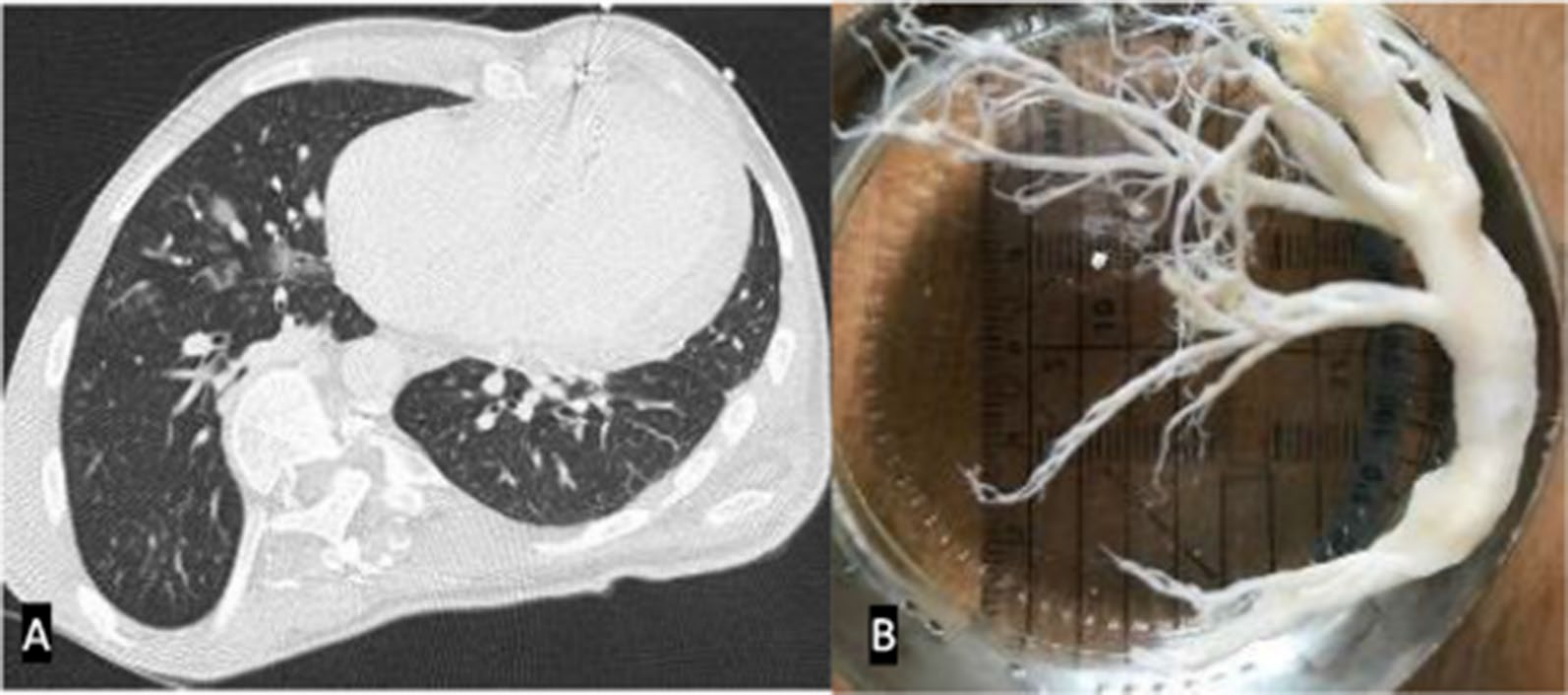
A CT Axial image in the lung window in a patient with plastic bronchitis. Note the groundglass parenchymal lung changes in the right upper lobe. B A bronchial cast retrieved in a patient with plastic bronchitis
